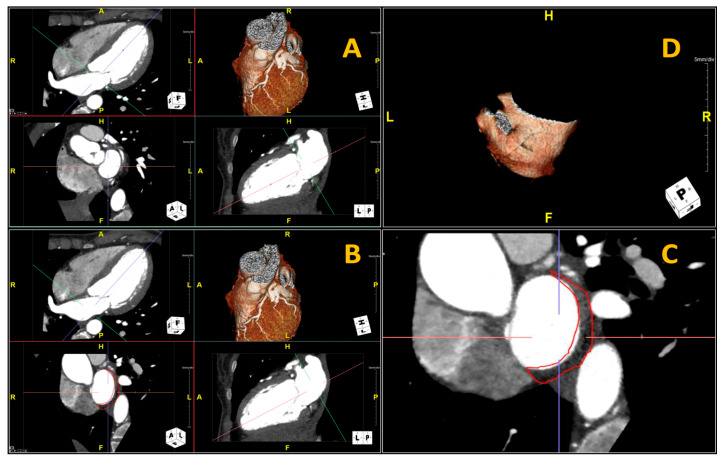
Reconstruction of the cross-sectional view of the left atrium, to measure the adipose tissue located posterior to the left atrium volume and attenuation on the cardiac computed tomography angiogram. (A) represents the first slice selected at the coronary sinus position and in (B) one slice in the mid-atrial position. (C) shows the manually traced region of interest (ROI) of a specific slice. (D) shows the reconstruction of three-dimensional ROI.
